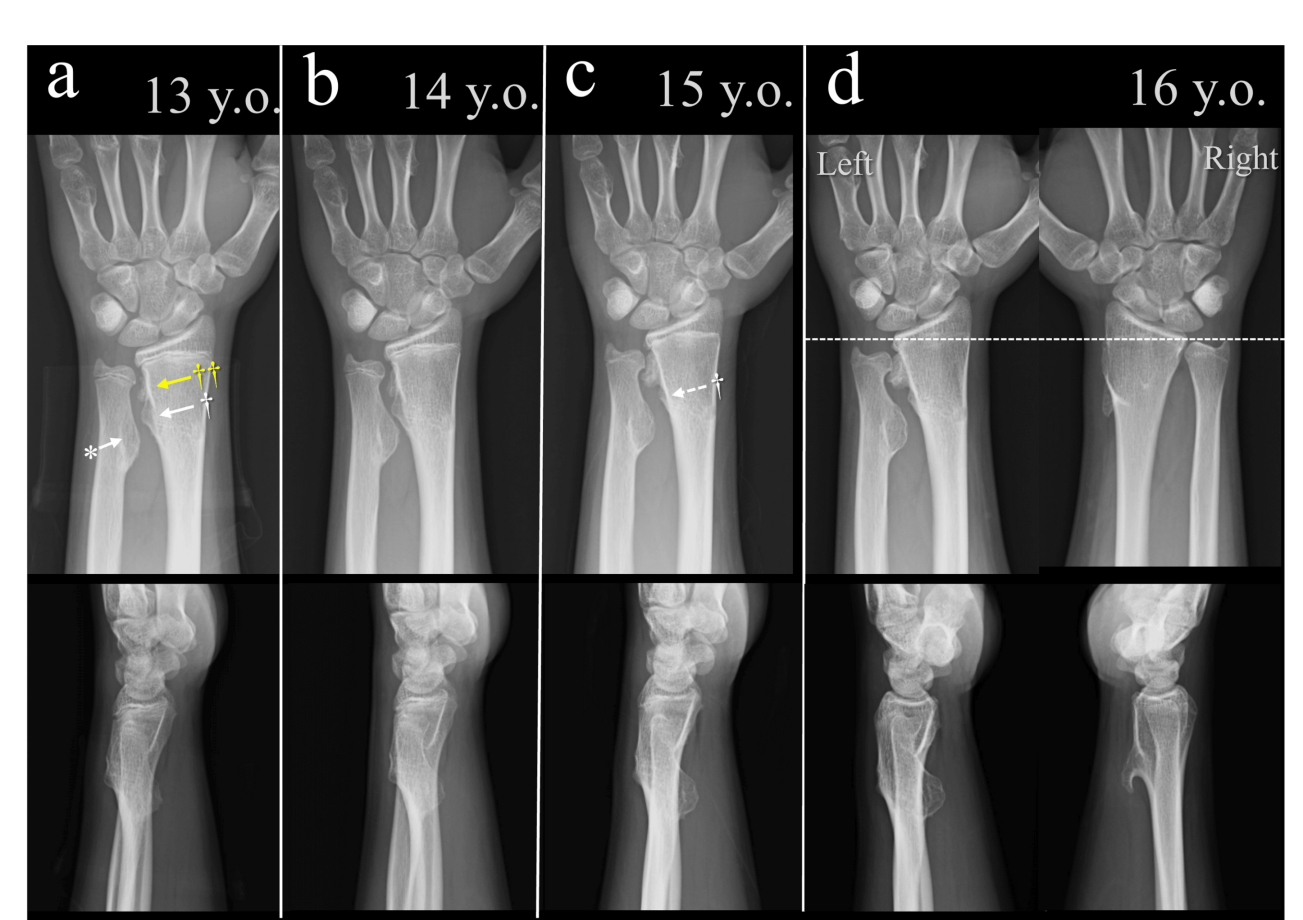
Serial radiographs of the left wrist from ages 13 to 16 (a) Age 13: A prominent ulnar osteochondroma is visible (asterisk), along with two sessile radial osteochondromas — proximal (dagger) and distal (double dagger, yellow). (b) Age 14: All lesions enlarged and became closely approximated. (c) Age 15: The proximal radial lesion regressed (dagger with dotted arrow), while the distal radial lesion continued to enlarge. (d) Age 16: The distal radial osteochondroma followed the contour of the ulnar head. Ulnar variance is shown with a white dotted line; the right wrist is included for comparison.
